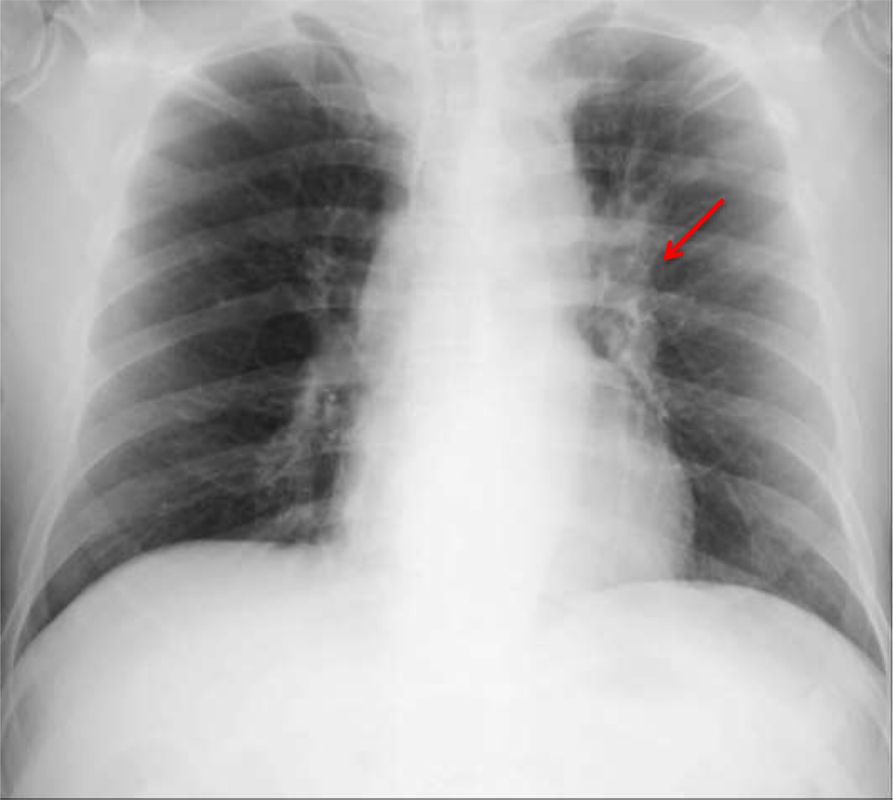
Chest x-ray revealing a left hilar mass (*red arrow*)
